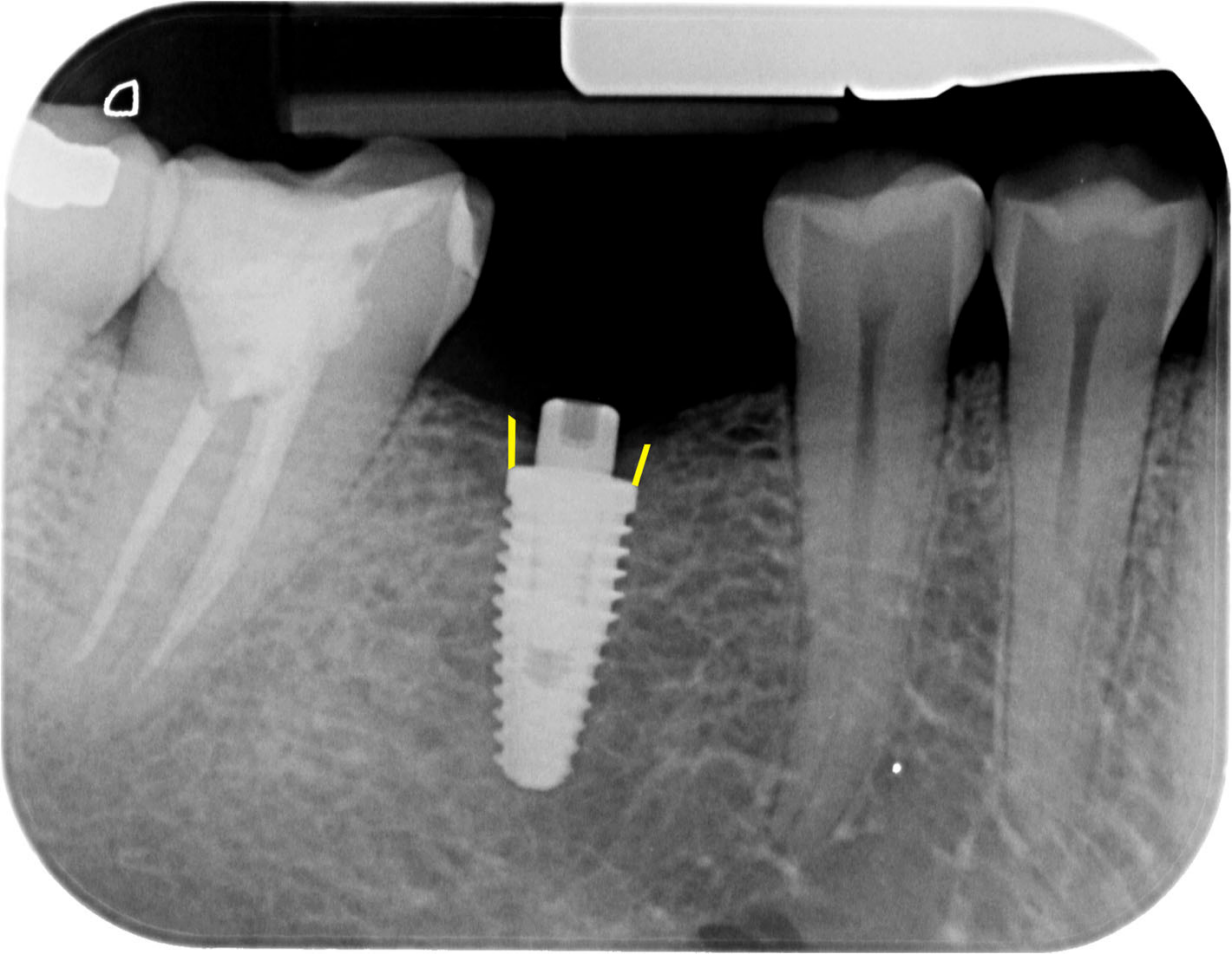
Representative image of the radiographical assessments. The distance between the implant platform and alveolar bone crest was measured both in mesial and distal regions, in millimeters, and averaged by each implant
